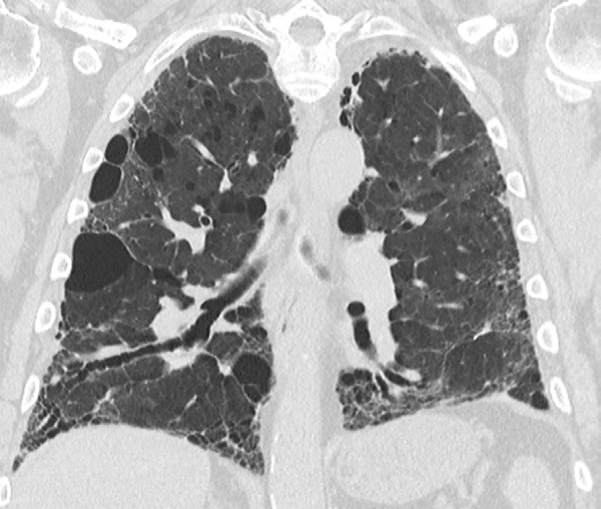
Combined pulmonary fibrosis and emphysema. Coronal CT image demonstrates upper lung–predominant centrilobular and paraseptal emphysema with additional lower lung, peripheral-predominant reticular abnormality, traction bronchiectasis, and honeycomb cyst formation.
